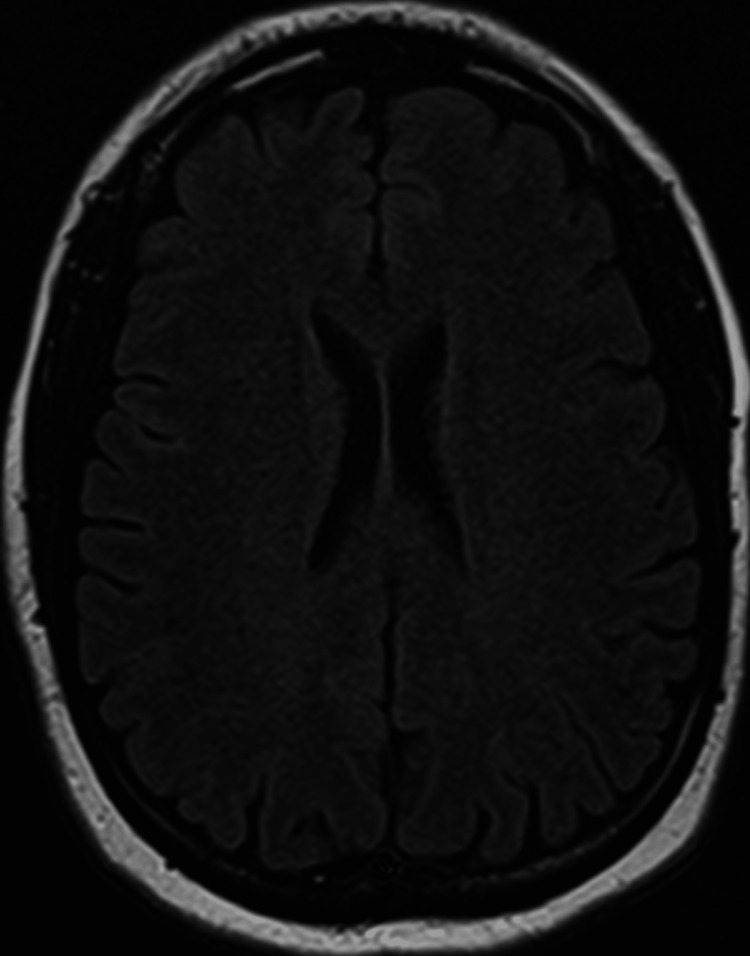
Brain MRI Axial Brain MRI showing no evidence for acute infarct in the basal ganglia. No mass or mass effect. No subdural hematoma.
